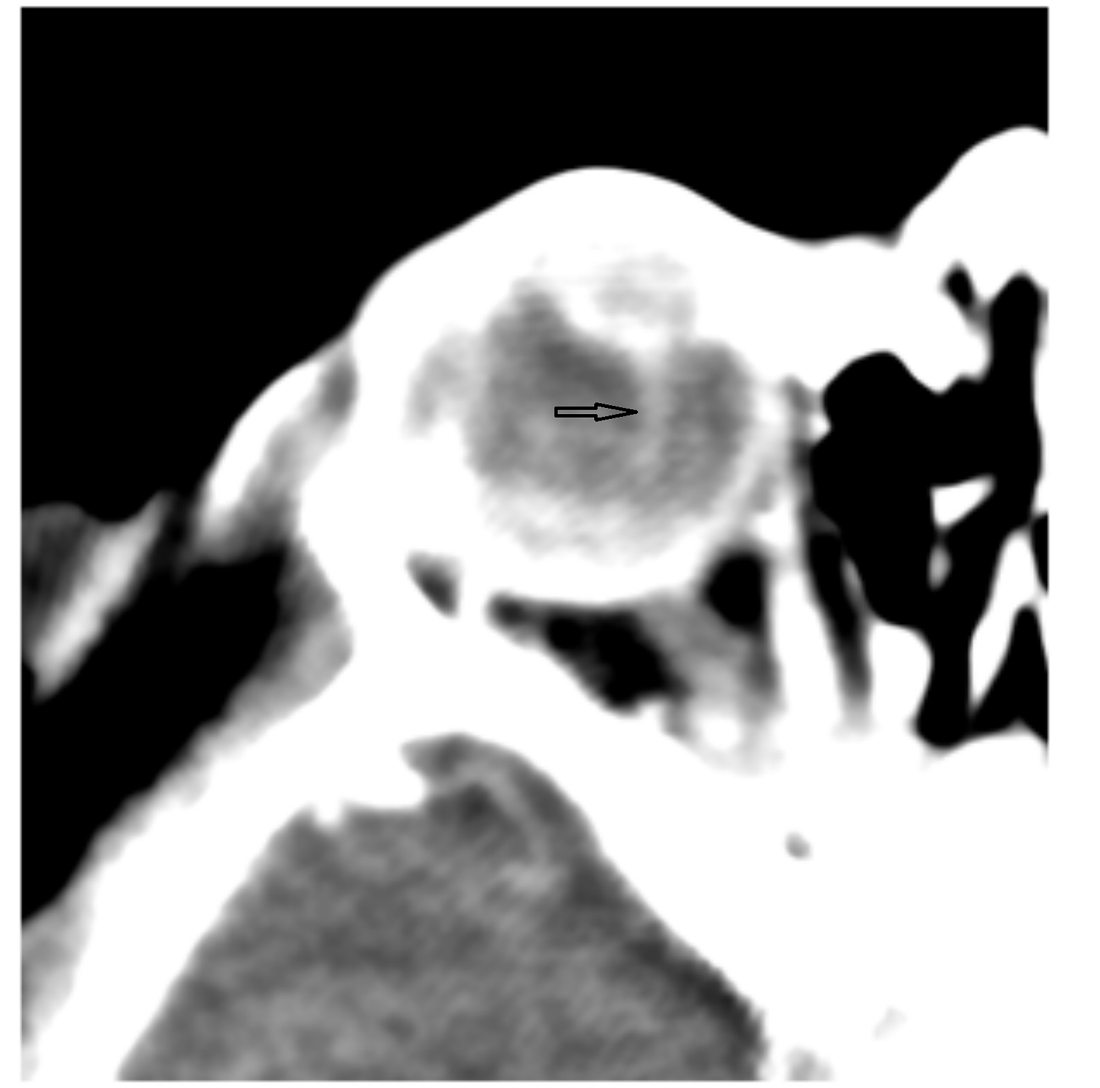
Post-contrast CT scan of the right orbit showing a thread-like extension (black arrow) in the posterior segment of the globe CT: computed tomography
